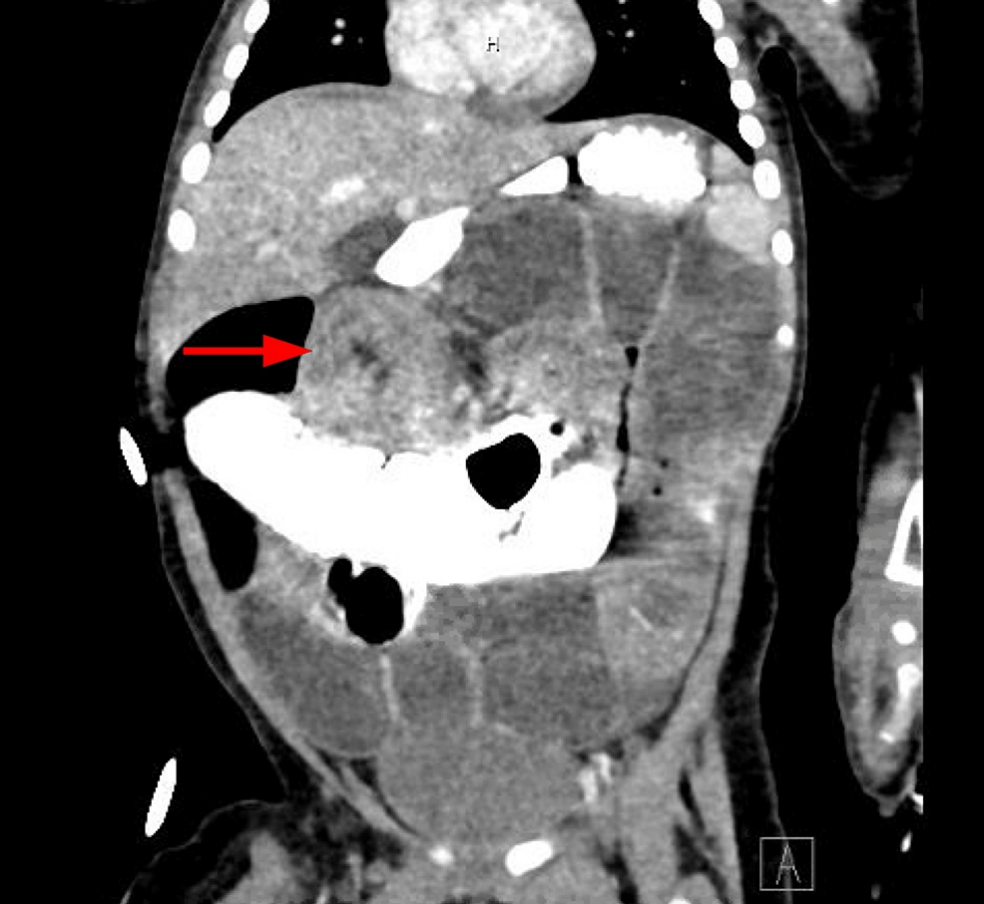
CT of the abdomen with contrast Concentric ring structure measuring 3.5 cm in size in the subhepatic region, concerning for ileocolic intussusception CT: computed tomography
